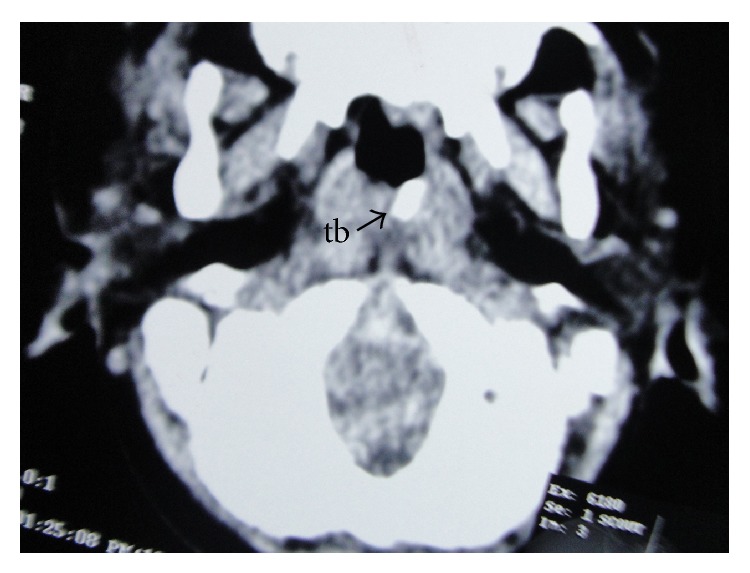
CT scan passing through the atlantooccipital joint, showing the handle of the toothbrush (tb) coming out to the surface from the retropharyngeal space of the nasopharynx.
